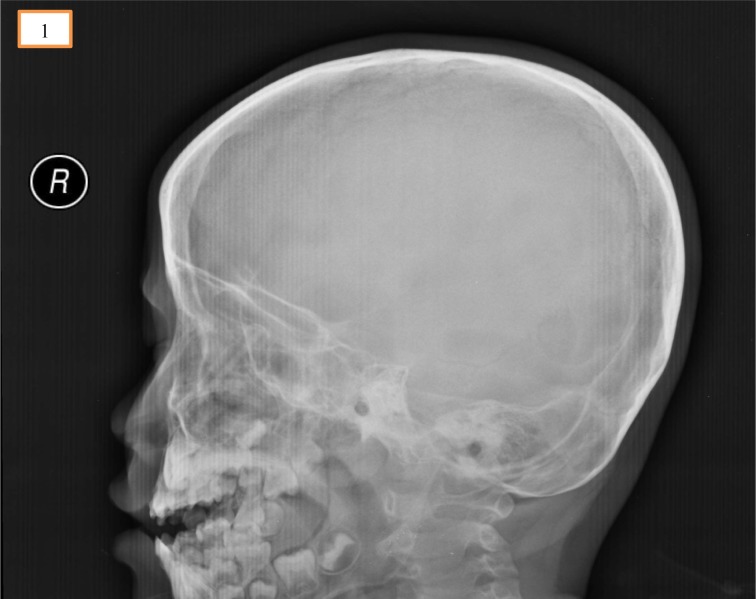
Radiograph of the skull showed faint pencil shadow over the left nasal cavity projecting towards the anterior skull base.
